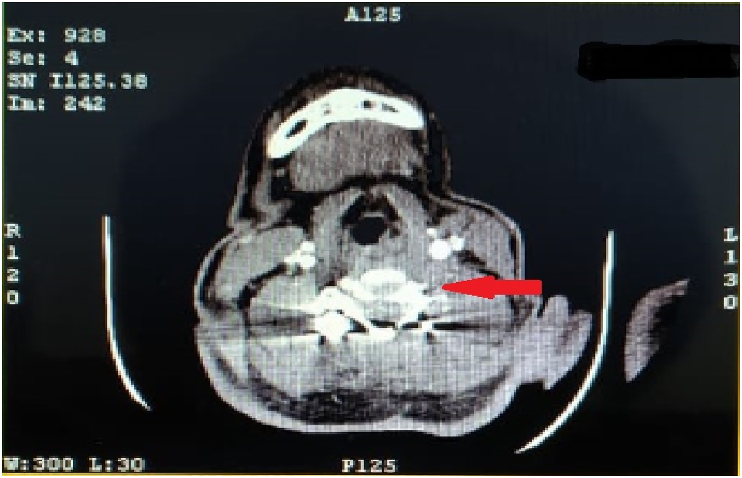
Post-operative CT angiography of the cervical depicting the unpresence of left vertebral artery (red arrow). (For interpretation of the references to colour in this figure legend, the reader is referred to the Web version of this article.)
